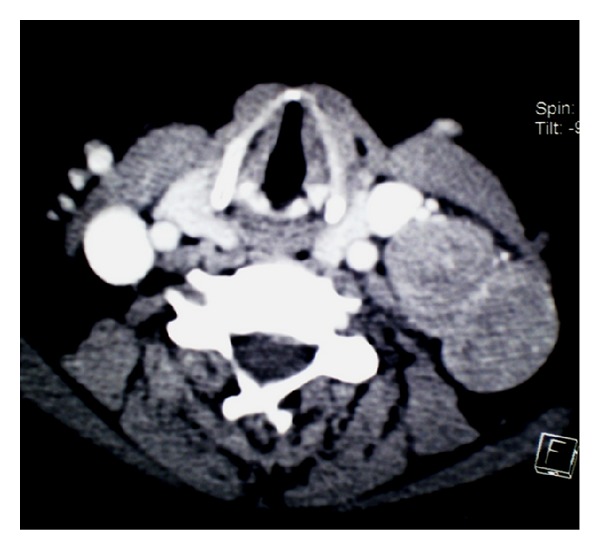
Contrast enhanced neck CT scan axial cup: left lateral jugular lymphadenopathy with heterogenic tissue density.
